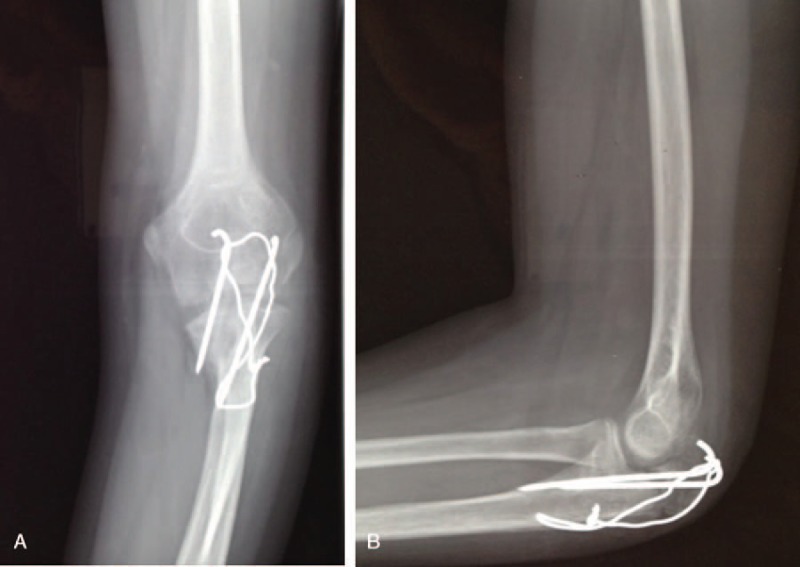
X-rays at 60 days after surgical treatment, performed with tension band wiring, in the anterior–posterior (A) and lateral (B) planes.
